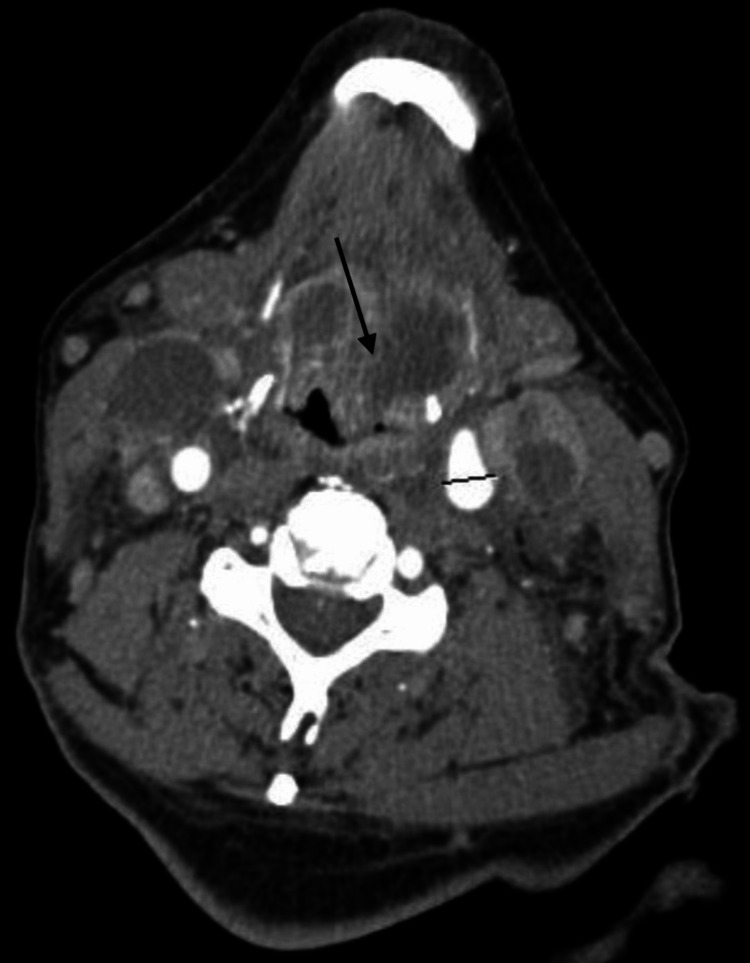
Bilateral, necrotic cervical lymph nodes on CT angiogram with IV contrast of neck. Necrotic tissue was noted in sublingual space, with some impingement of hypopharynx (arrow) before tracheostomy placement.
